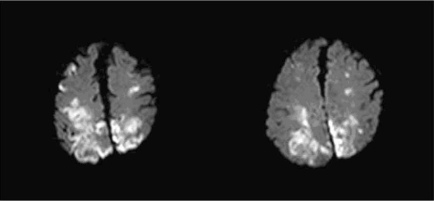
MRI Diffusion-weighted image (DWI) showing bilateral infarcts in MCA and ACA territories suggestive of embolic origin.
